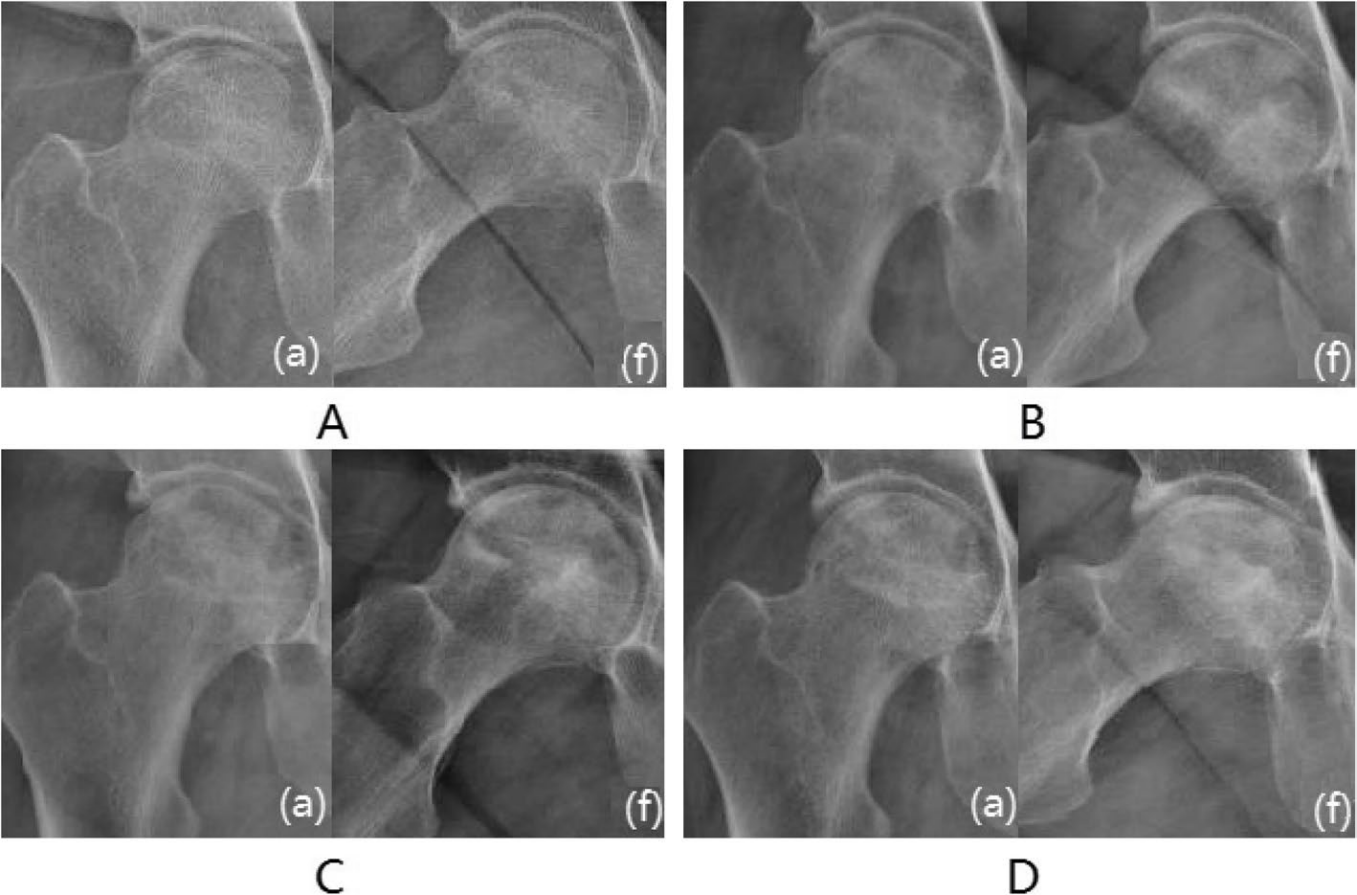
Typical case 1: Male, 45 years, with right hip pain for more than 8 months. A Radiograph imaging at an anteroposterior (a) and frog (f) lateral view. The patient's CSI, BSI and ISI were 6.36,2.36 and 0.53,respectively. B Six months. C 1 year. D After 2 years, the femoral head did not collapse significantly, and the density of the necrotic area increased
